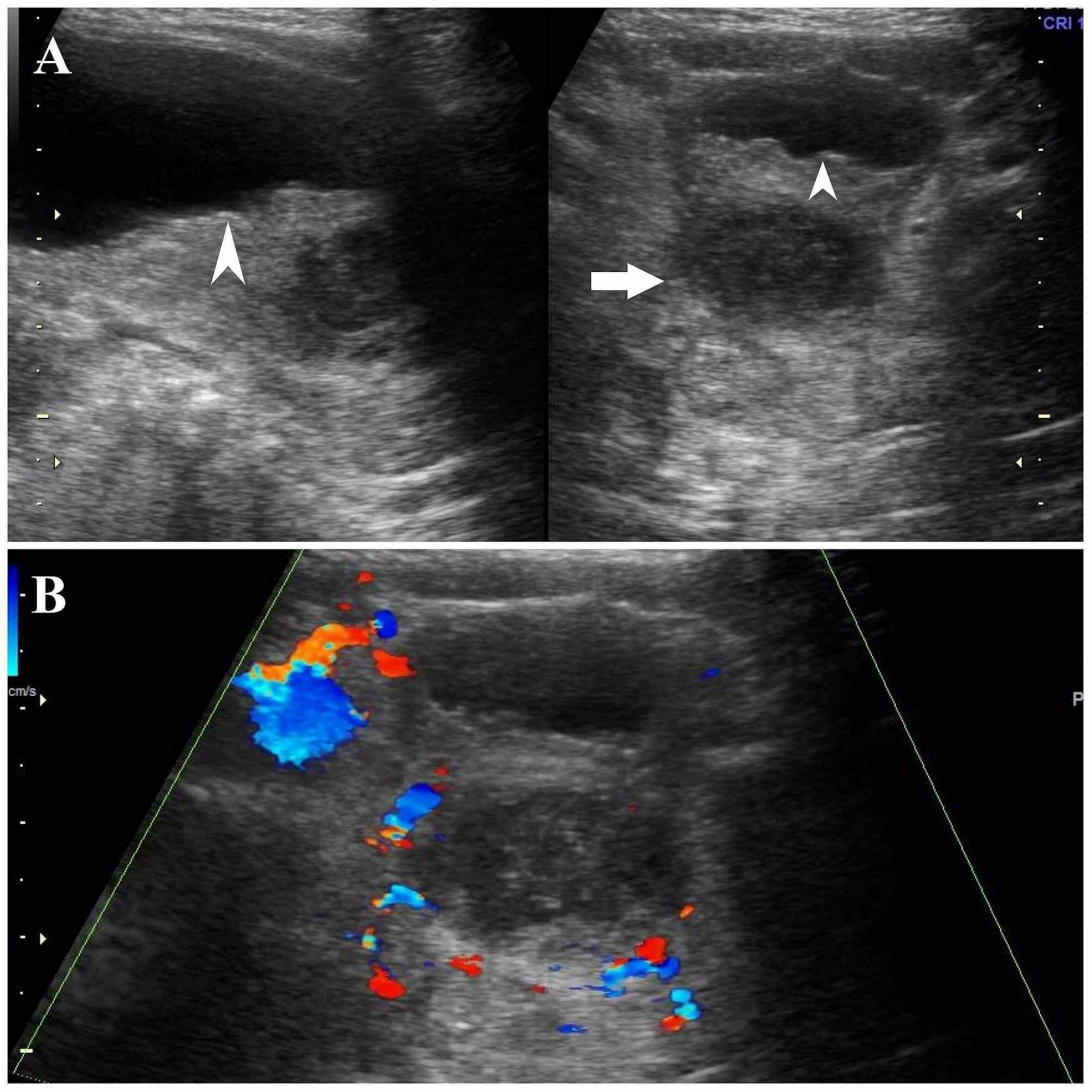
Ultrasound of the urinary bladder and prostate with Doppler imaging A – Arrowheads show prominent wall thickening, and mucosal irregularities are seen along the inferior wall of the urinary bladder. The horizontal arrow shows the enlarged hypoechoic appearance of the prostate gland. B – Colour Doppler imaging of the prostate shows prominent peripheral vascularity with no definite central vascularity.
